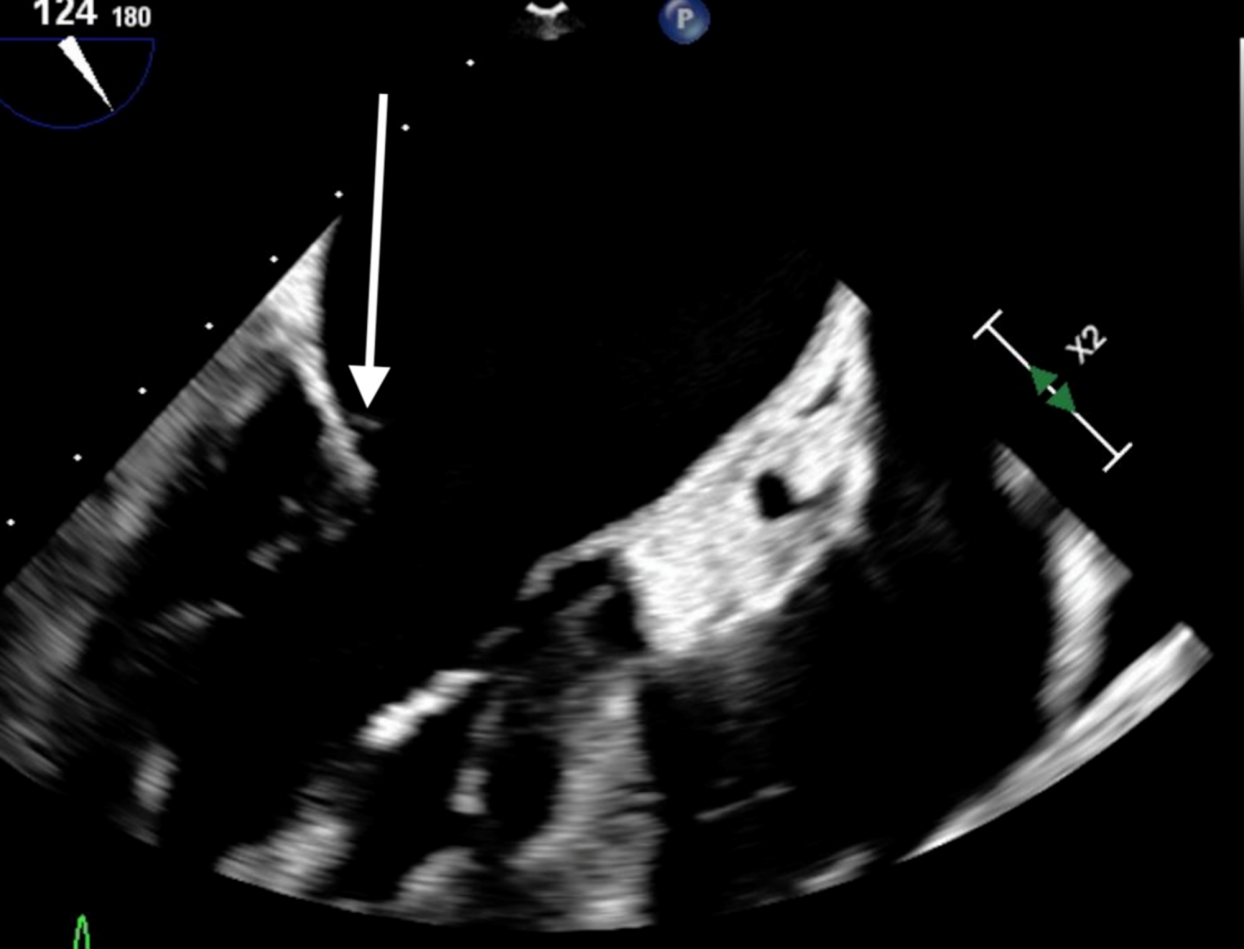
Transesophageal echocardiogram showing vegetation on mitral valve
